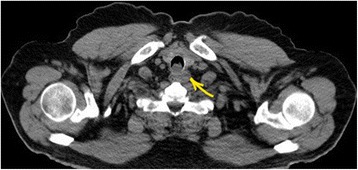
CT scan of the chest showing dumbbell shape endotracheal tumor with extratracheal extension
